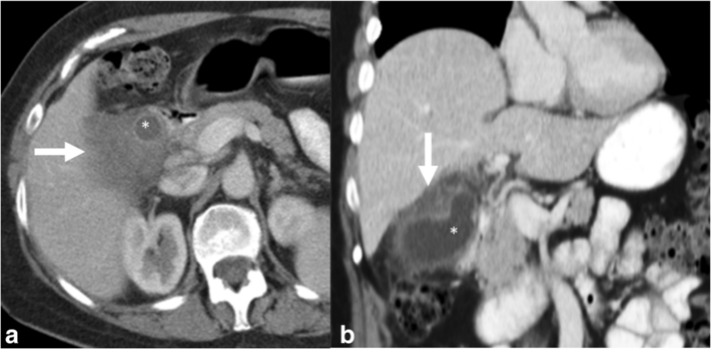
Gall bladder carcinoma. Contrast enhanced axial CT image (a) and coronal reformat (b) showing hypodense thickening of the gall bladder wall representing the carcinoma (arrow) with involvement of the adjacent liver. The thickening covers more than half of gall bladder lumen (*).
